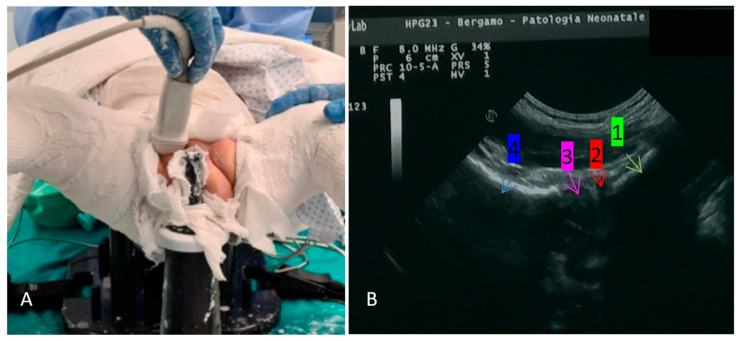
(A) The patient is in narcosis on a pediatric table, the SC has already been performed (in human position, according to Fettweis); the right hip is checked with TIT, through the same perineal opening of the cast used for wipers; and (B) the sonographic image obtained in the same patient after reduction: 1 ramus superior os pubis, 2 labrum, 3 femoral head, 4 femoral neck.
